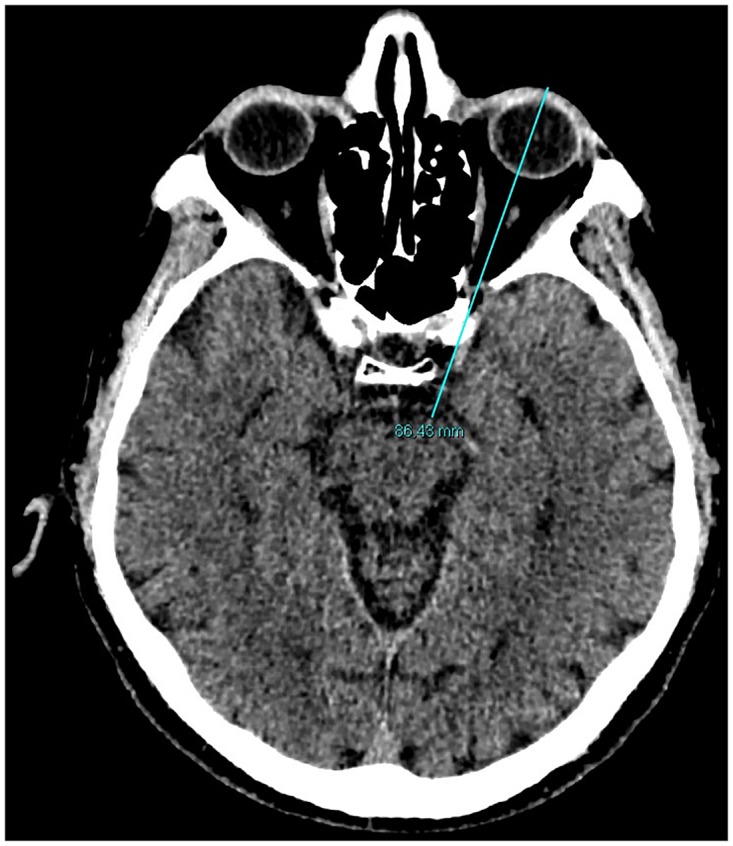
Measurements of the distances using linear system on axial CT scan. The distance between the ocular skin and the substantia nigra (D-SS).
